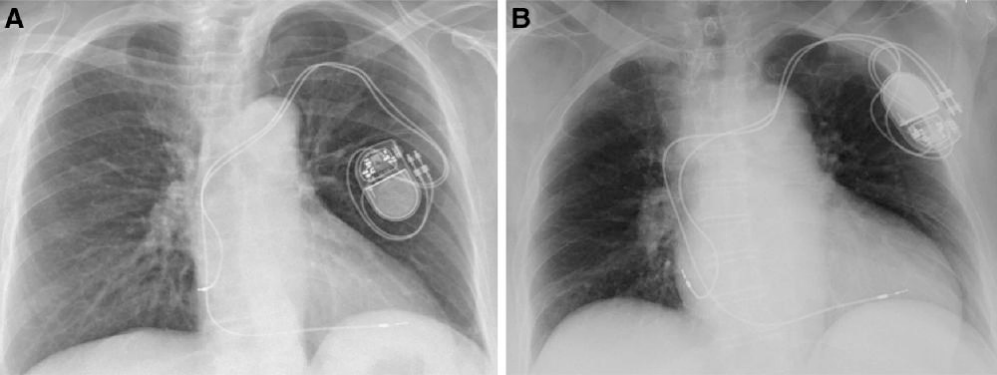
Case report 2. (*A*) Post-implant chest X-ray. (*B*) Chest X-ray—1 month later.
